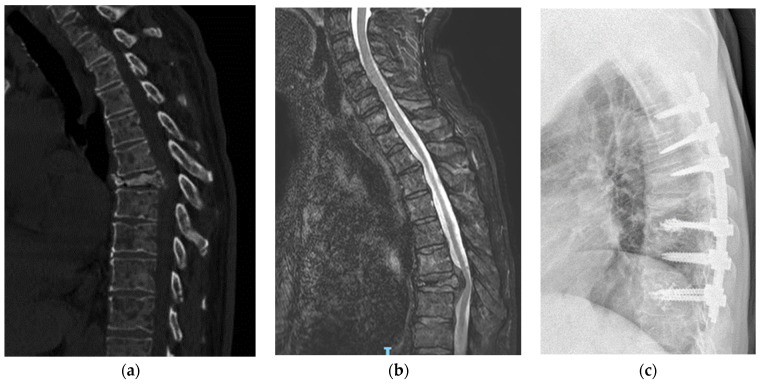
Case of percutaneous stabilization. A 83-year-old male, affected by hepatocarcinoma for 4 years, undergoing chemotherapy. During regular follow-up, CT scan image (a) showed T7 fracture. The patient presents other non-spinal multiple osteolytic lesions. In MRI image (b), mild compression is found. The patient was asymptomatic except for dorsal pain. Further analysis showed multiple lung and bone metastases. According to clinical symptoms, clinical condition and prognosis of the patients, we performed percutaneous screw fixation with augmented screws (c).
